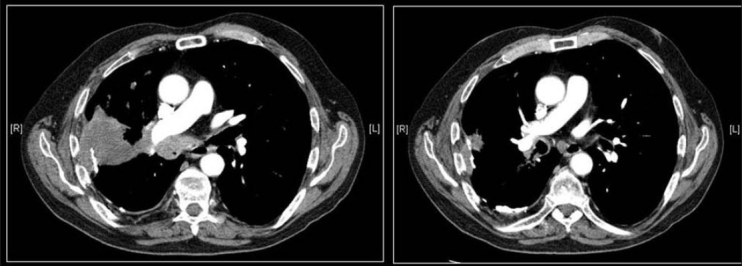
Axial CT scans of the same patient as in Figure 13 shows a pleural-based mass with underlying pleural calcification. A CT-guided biopsy revealed a small cell lung cancer
